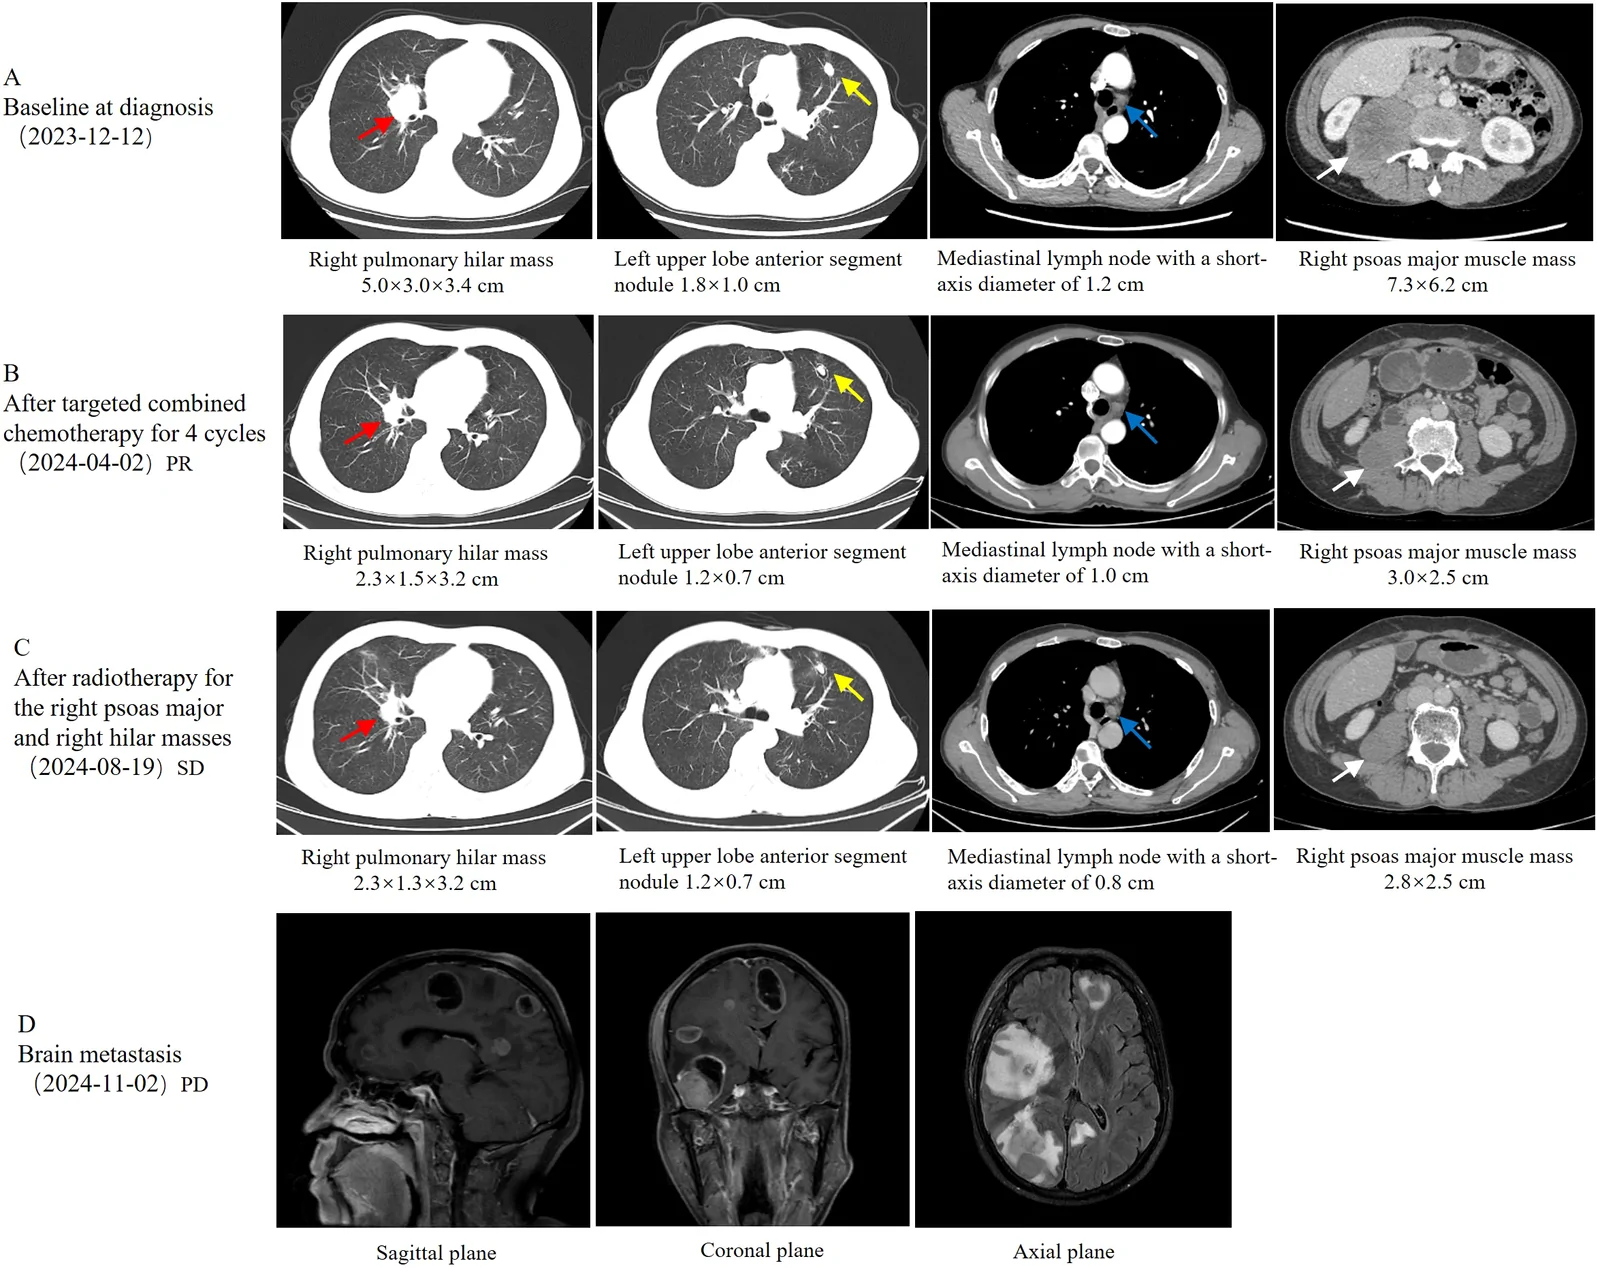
Imaging results. (A-C) Chest and abdominal CT images. Right pulmonary hilar mass (red arrow). Left upper lobe anterior segment nodule (yellow arrow). Mediastinal lymph node (blue arrow). Right psoas major muscle mass (white arrow). (D) Brain MRI showing multiple intracranial metastases.
